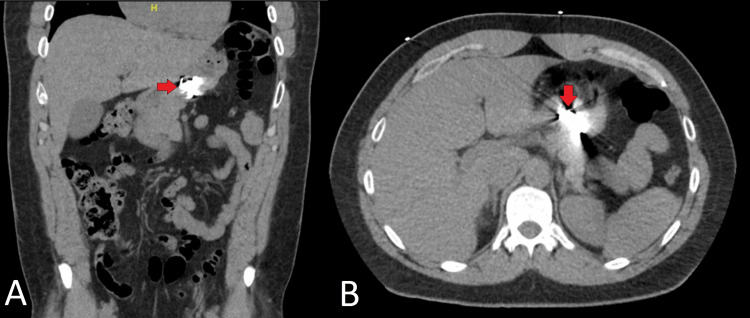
Computed tomography scan of foreign body ingestion (see arrow) Coronal (A) and axial (B) views of the abdomen and pelvis revealing a high-density foreign object in the gastric body.
